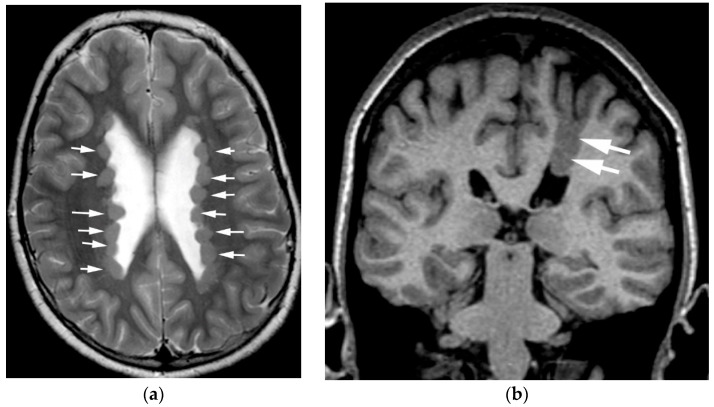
(a) Numerous periventricular nodular heterotopia (white arrows) are seen on this axial T2-weighted image. Note how they have the same signal intensity as cortex. (b) Transmantle grey matter heterotopia (white arrows) extending from the left superior frontal gyrus to the body of the left lateral ventricle seen on this coronal T1-weighted image.
